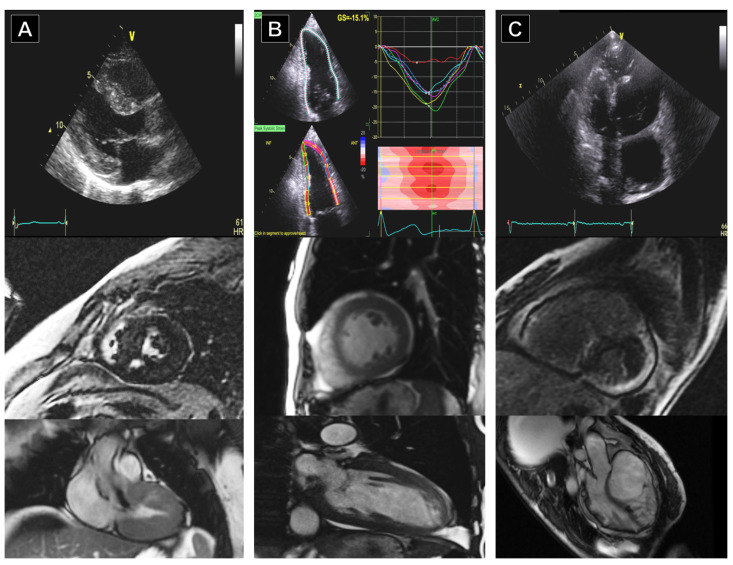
Patient 1 (A): A patient with left ventricular hypertrophy in transthoracic echocardiography who exhibits delayed gadolinium enhancement in the region of the anterior septum on CMR. Patchy enhancement is focal, with another tiny enhancement seen along the base in the mid-septum, compatible with HCM. Patient 2 (B): A patient with increased left ventricle diameters and mildly reduced systolic function in echocardiography. CMR findings were consistent with dilated, nonischemic cardiomyopathy, confirming the left ventricle dilatation and decreased global systolic dysfunction. Patient 3 (C): A patient with ARVC who showed severe right ventricle and right atria dilation with diminished right ventricle function. Extensive sacculation, right ventricle hypertrophy, and enlargement are consistent with the diagnosis of ARVC. The extensive LGE along the right ventricle free wall and right-sided interventricular septum further support this diagnosis.
